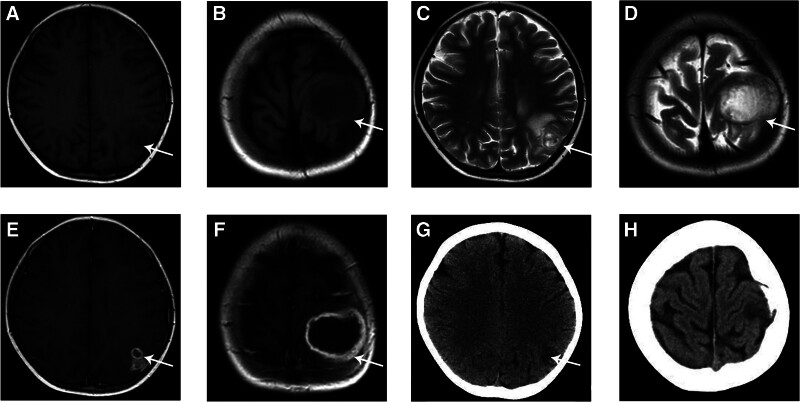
Head MRI (A, B) showed multiple longer T1 in the left parietal lobe. (C, D) showed multiple longer T2 in the left parietal lobe. (E, F) The enhanced scan showed multiple abnormal signal lesions in the left parietal lobe with circular enhancement-the possibility of abscess formation. Head CT (G, H) 1 month after discharge showed that the lesions were more significantly reduced. CT = computed tomography.
